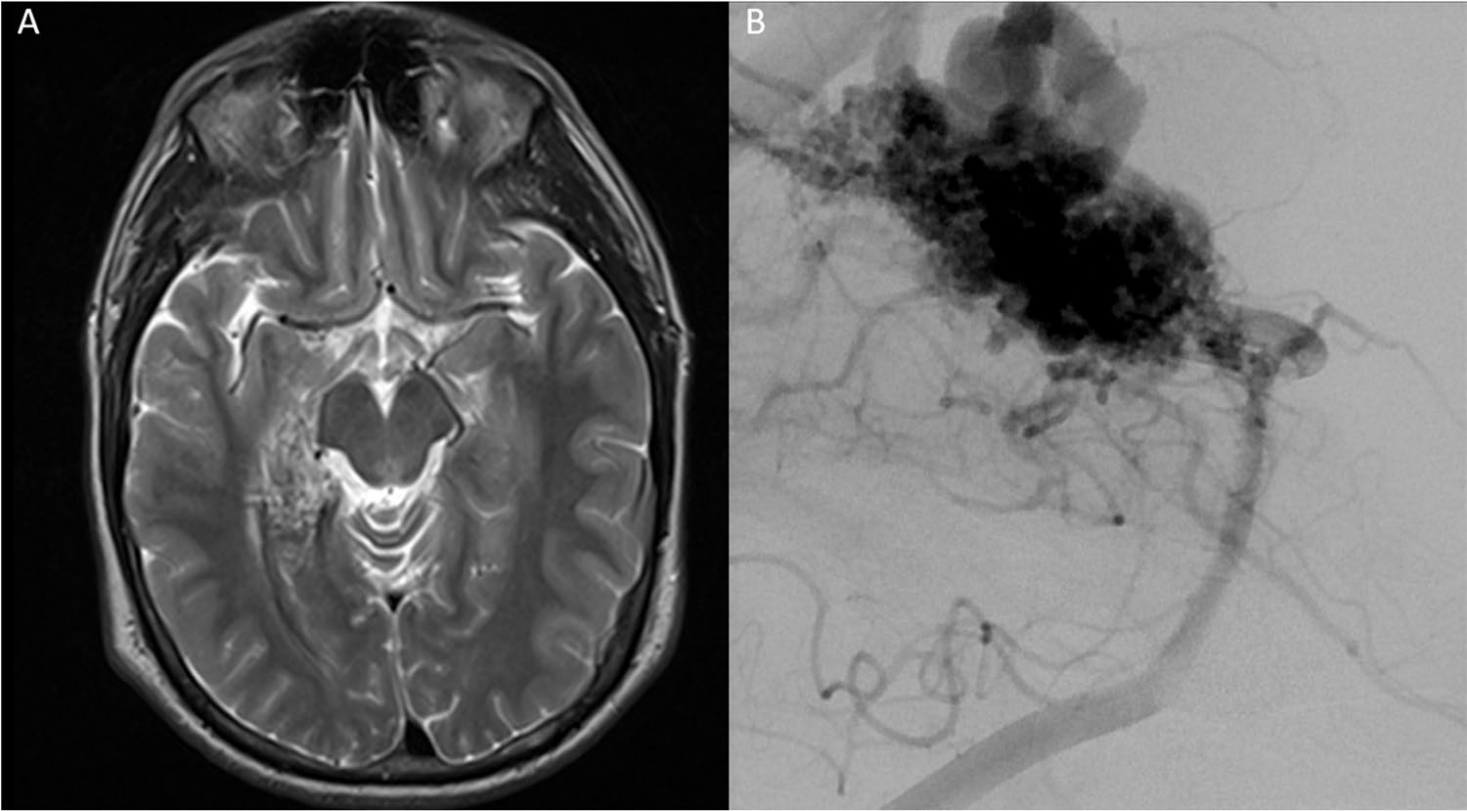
Axial MRI showing an AVM in the right temporal lobe measuring 37.5 mm × 17.4 mm × 32 mm. Lateral view of vertebral injection depicting AVM with deep venous drainage and intranidal aneurysms
